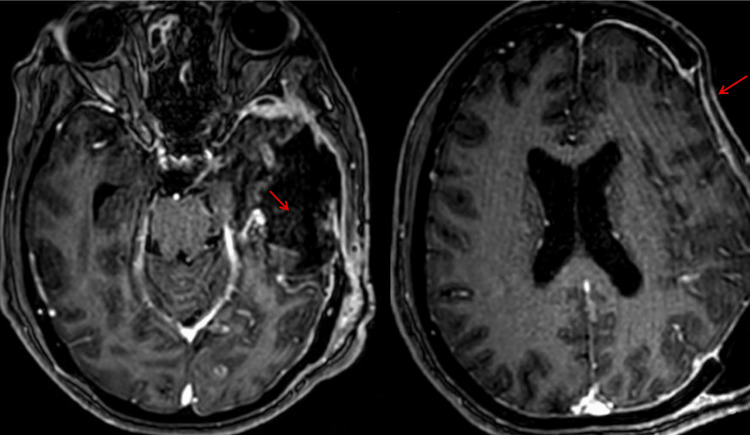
Follow-up brain magnetic resonance imaging (simple and contrast) showing postoperative changes with no residual injury Follow-up axial brain magnetic resonance imaging demonstrating postoperative changes in the left fronto-temporo-parietal region, with a post-surgical cavity and mild linear peripheral enhancement, without evidence of residual lesion, recurrent hemorrhage, abnormal mass enhancement, or midline shift (red arrows).
